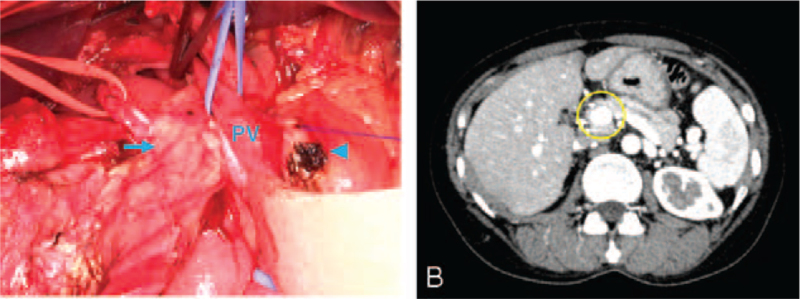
Computed tomography and intraoperative findings in case 2. (A) Following pancreatic transection, the dorsal (blue arrow) and ventral (blue arrowhead) margins of the portal annular pancreas can be observed. (B) The image shows the portal annular pancreas (yellow circle). PV = portal vein.
